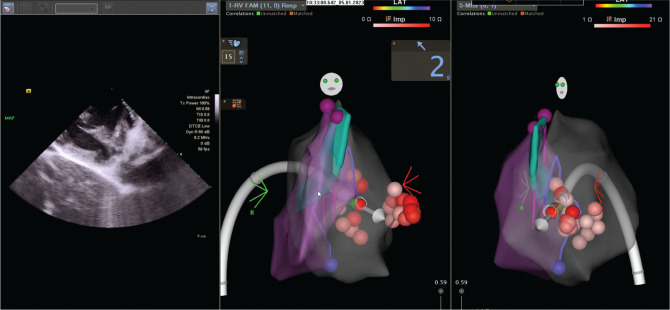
Intracardiac echocardiography image and electroanatomic map showing the ablation catheter in contact with the moderator band.
